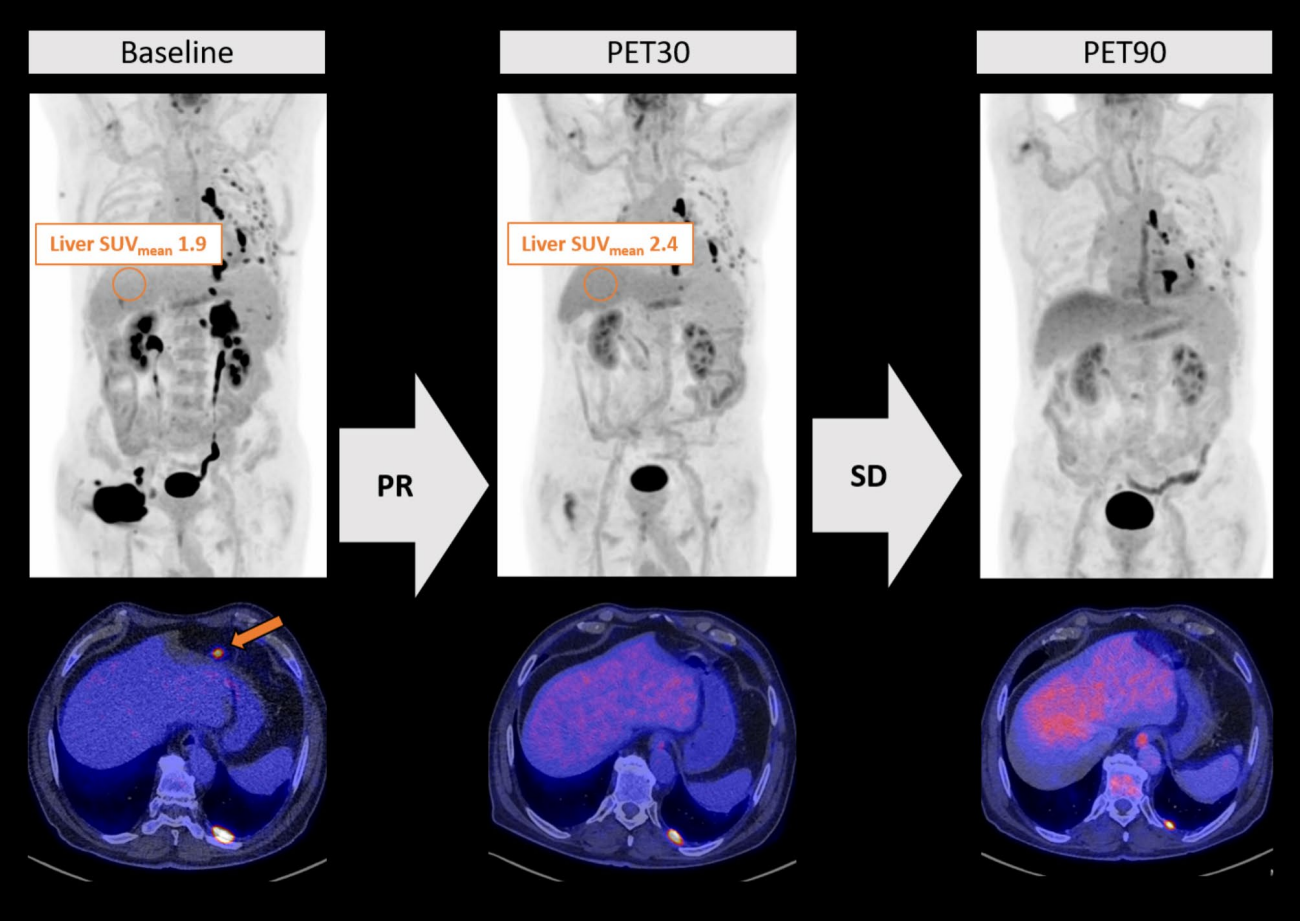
Increase in Liver-SUV mean is associated with longer disease control after CAR-T. Representative PET/CT images of in relapse depicting lymphoma at baseline (first column) with FDG-avid lymphonodale manifestations in the abdomen and the right inguinal region. Furthermore PET/CT presents with highly metabolic active pleural lesions of unclear origin mostly associated with pleurodesis but lymphoma could not be ruled out entirely. PET30 after CAR-T (second column) shows decreasing metabolic activity of the lymphoma with remaining FDG-avid pleural lesions overall rated as partial remission. Representative measurements of the Liver-SUV_mean_ at Baseline and PET30 are shown in the upper row. PET90 images (third column) depicts stable disease with multiple FDG-avid pleural lesions. Two years after CAR-T the patients remains in disease remission. CD19-CAR-T cell therapy (CAR-T), Fluor-18-Deoxyglucose (FDG), PET/CT scan at day 30 (PET30), PET/CT scan at day 90 (PET90)
